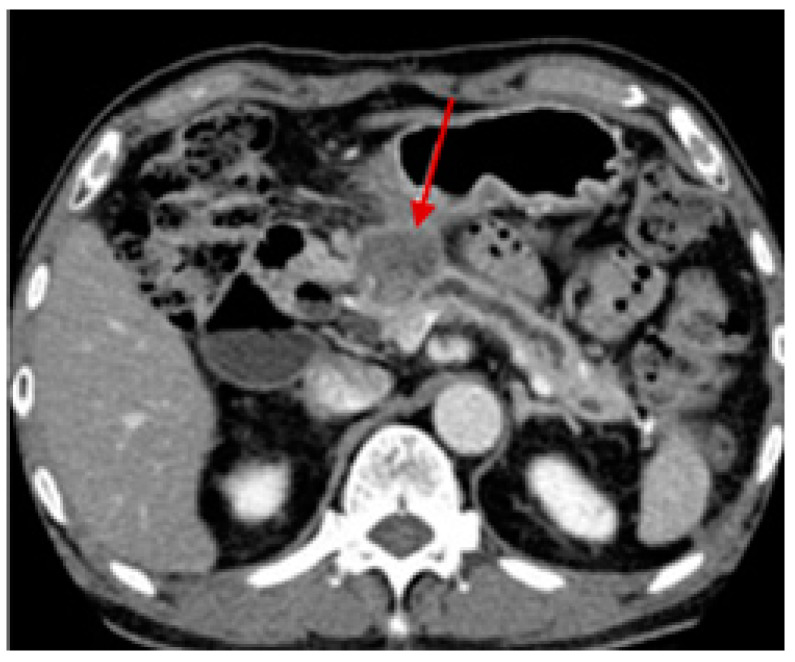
Computed tomography scan of a 73-year-old male patient with pancreatic cancer in the pancreas head with about 3 cm (arrow). Reprinted with permission from [65].
